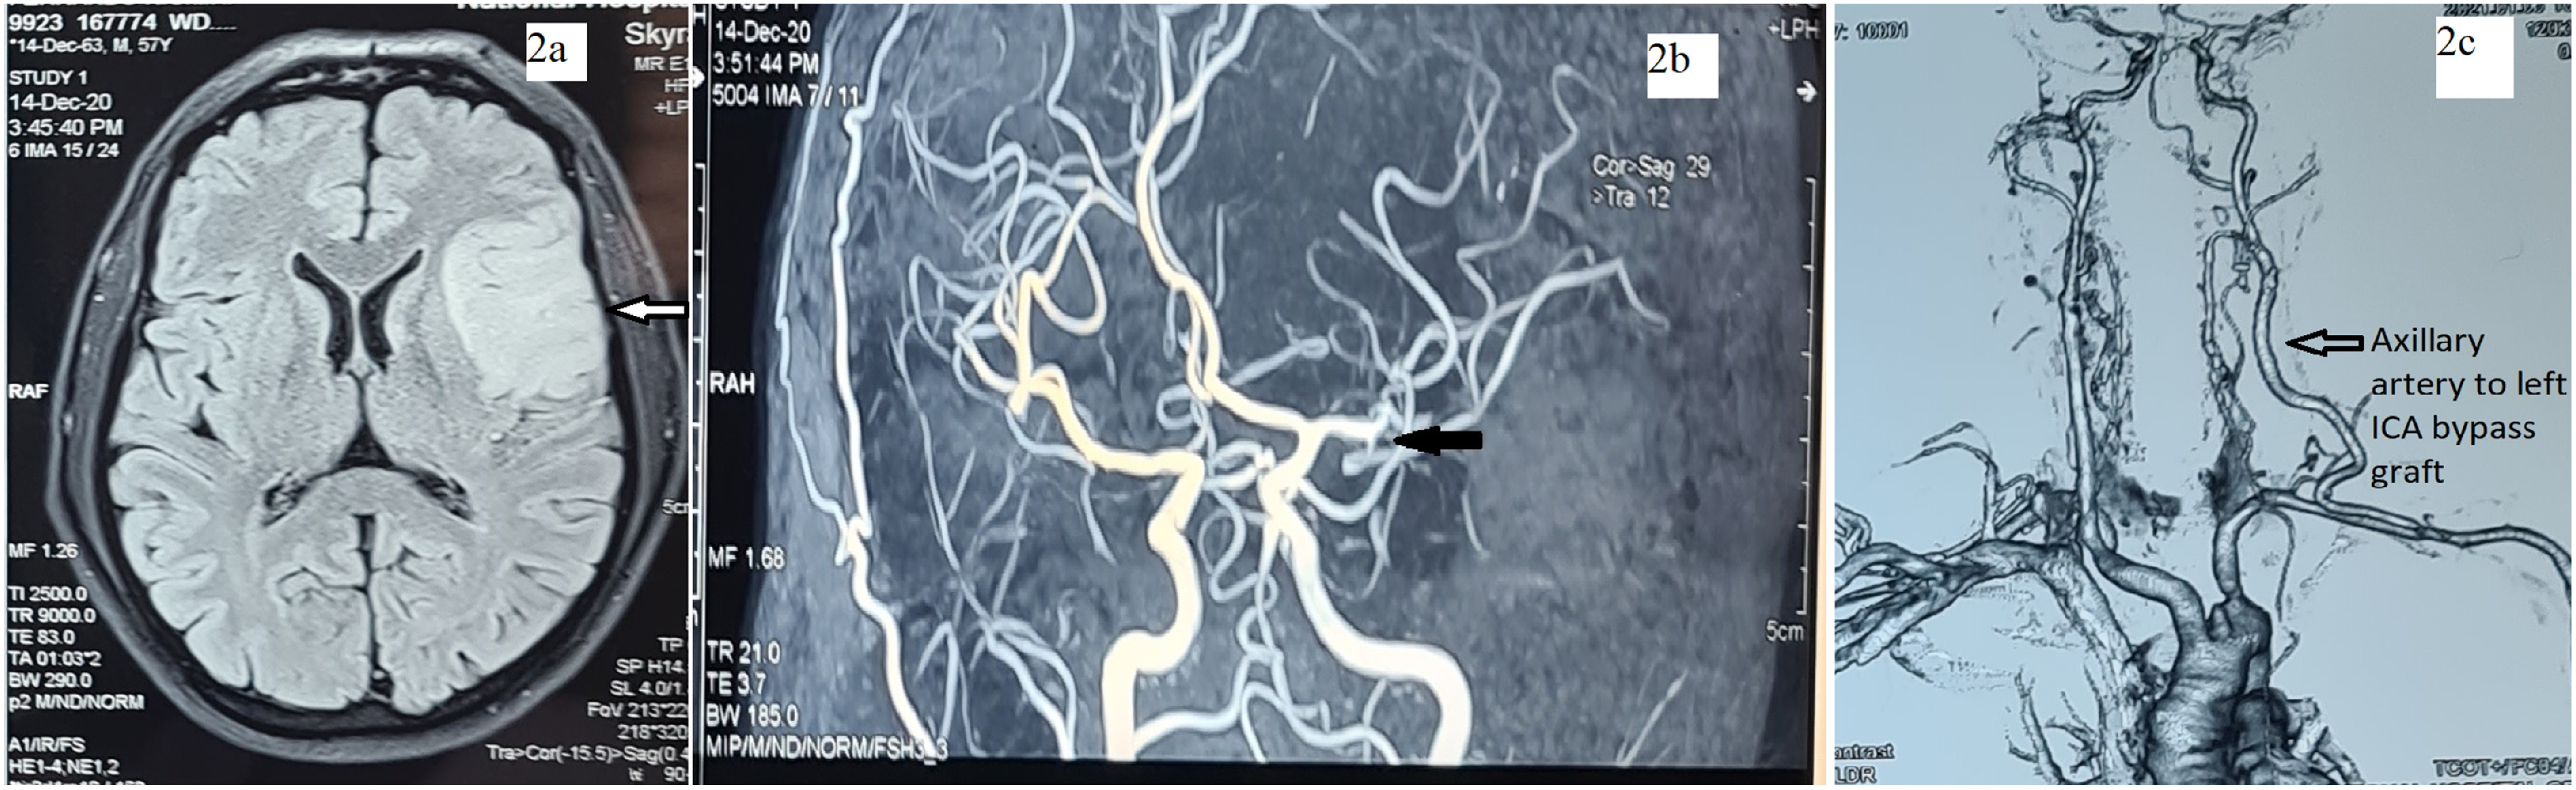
Fig. 2 (a) Magnetic resonance imaging showing the acute post-operative middle cerebral artery (MCA) territory infarction (arrow), (b) Magnetic resonance angiography of the occluded MCA (arrow), and (c) patent bypass graft on computed tomography angiogram (arrow).
